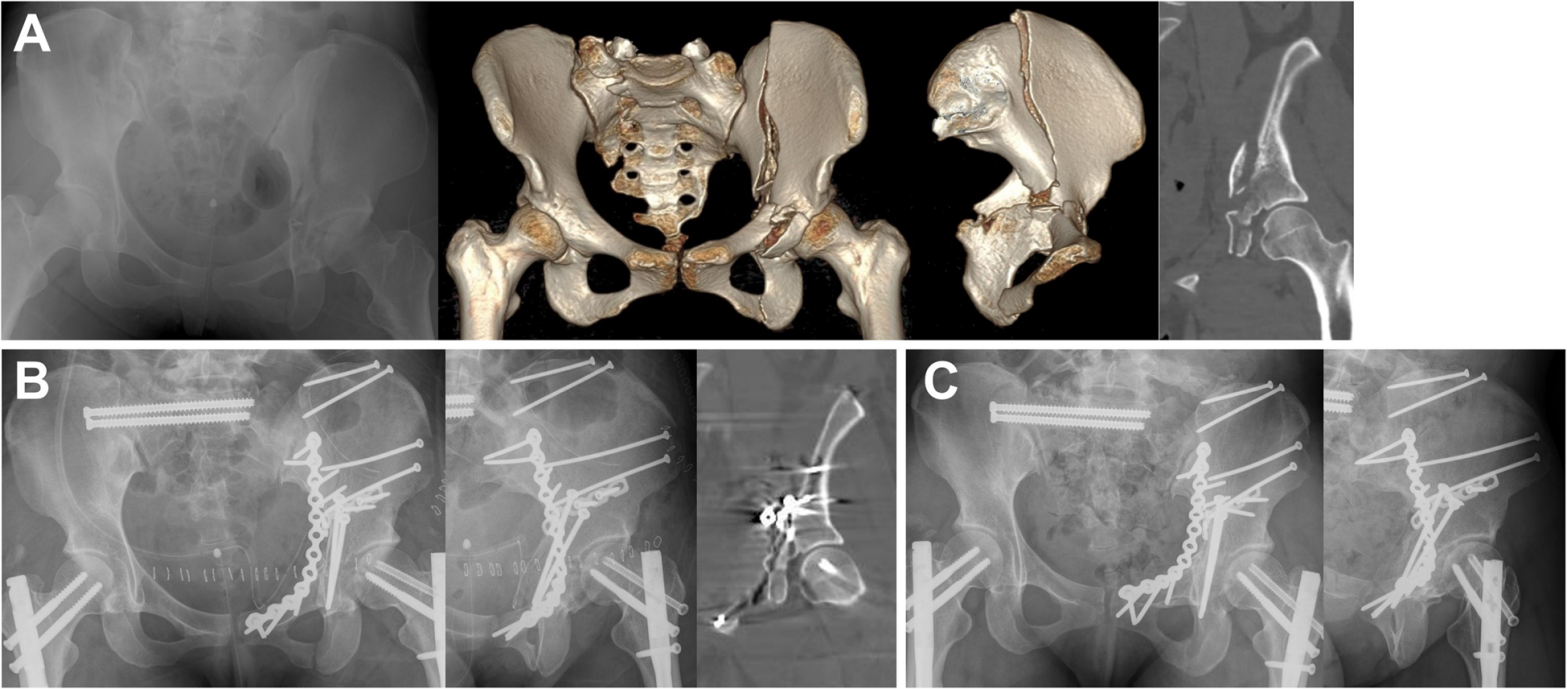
A 23-year old woman attempted suicide by jumping from a height. a The patient sustained a T-type fracture of the acetabulum without dome involvement and displaced pelvic ring injury. b Postoperative radiographs and coronal view of CT scan after open reduction and internal fixation for the acetabular fracture and pelvic ring injury are shown. The fractures were reduced and fixed via the anterior intrapelvic approach due to the associated iliac bone fracture and no involvement of the posterior wall. c Radiographs at 12 months after surgery show bony union and no evidence of arthritic change
